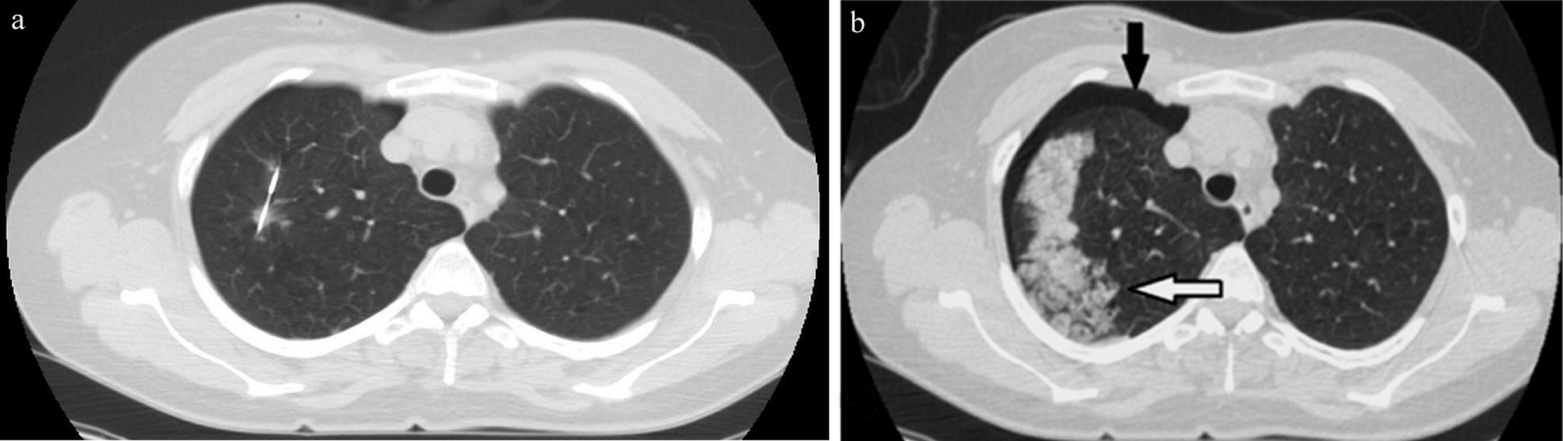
Complications of CT-guided lung biopsy. (a) CT-guided lung biopsy with a non-coaxial semi-automated 18 gauge biopsy system of a 9mm right upper lobe nodule which was proved to be bronchogenic carcinoma at pathologic examination. (b) Post-biopsy CT image showed complications in the form of a small pneumothorax 1cm (black arrow), and a new ground-glass opacity along needle tract measuring >2cm in width but sublobar corresponding to grade 2 pulmonary hemorrhage (white arrow).
